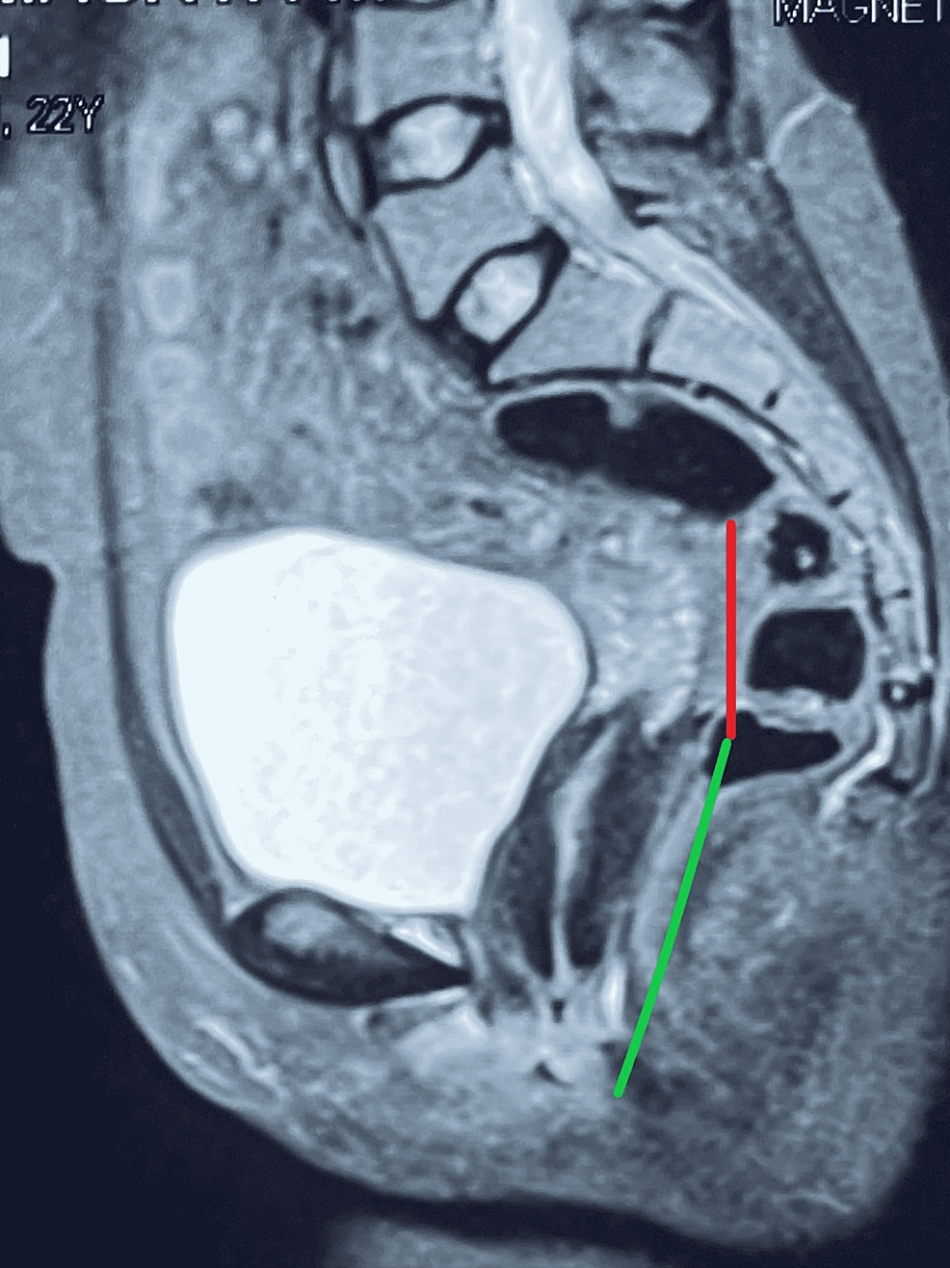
MRI pelvis showing a normal-sized uterus and an elongated cervix denoted by red and green lines, respectively.
